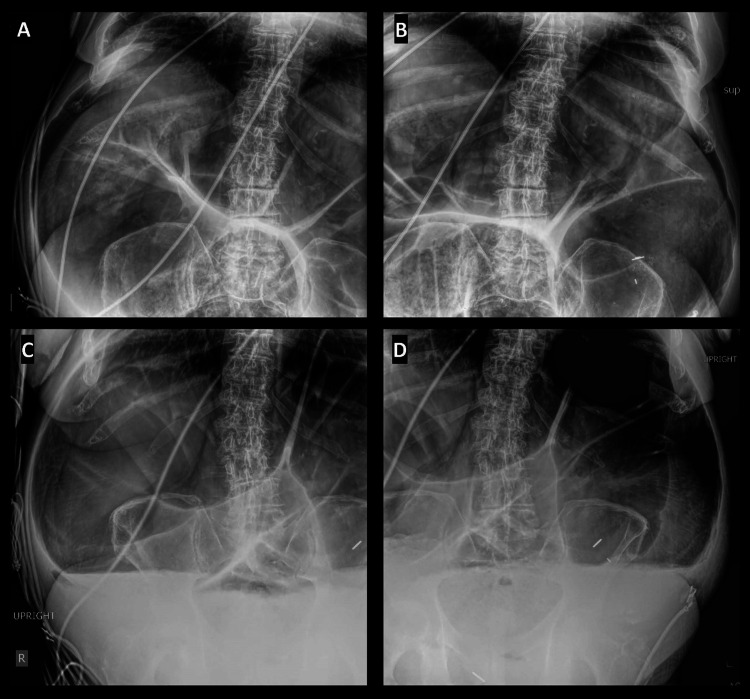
Abdominal series x-ray demonstrating extensively distended bowel (A) Right supine view. (B) Left supine view. (C) Right upright view. (D) Left upright view.
